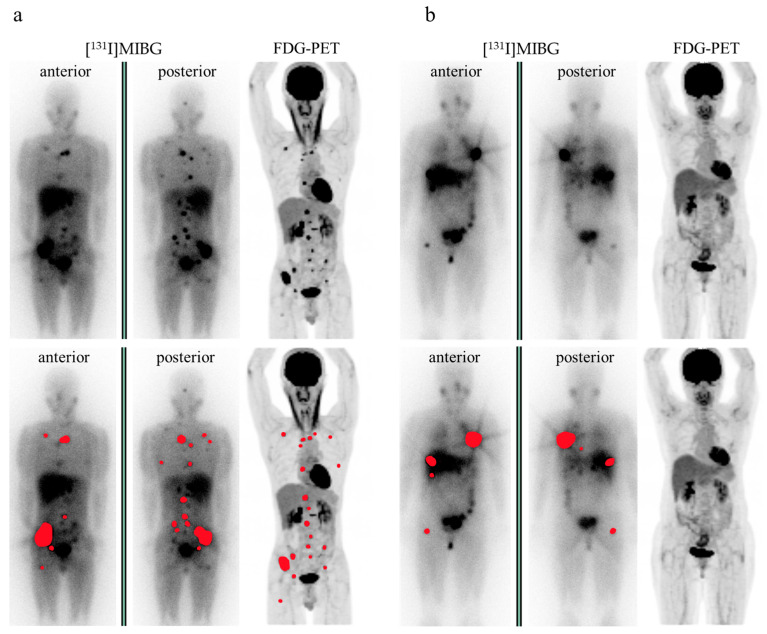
Representative cases. (a) A patient with bone and lymph node metastases. The treatment-dose [^131^I]MIBG scintigraphy (planner image) and the FDG-PET (maximum intensity projection [MIP] image) are shown. Metastatic lesions are indicated in red. Urinary catecholamine levels were as follows: adrenaline, 15.6 μg/day; noradrenaline, 3991 μg/day; and dopamine, 906.1 μg/day. All metastatic lesions demonstrated marked FDG avidity. (b) A patient with bone, liver, and lung metastases. The planner image of [^131^I]MIBG scintigraphy and the MIP image of FDG-PET are shown. Metastatic lesions are highlighted in red. Urinary catecholamine levels were as follows: adrenaline, 18.3 μg/day; noradrenaline, 179 μg/day; and dopamine, 963.2 μg/day. None of the metastatic lesions showed significant FDG uptake. MIP, maximum intensity projection.
